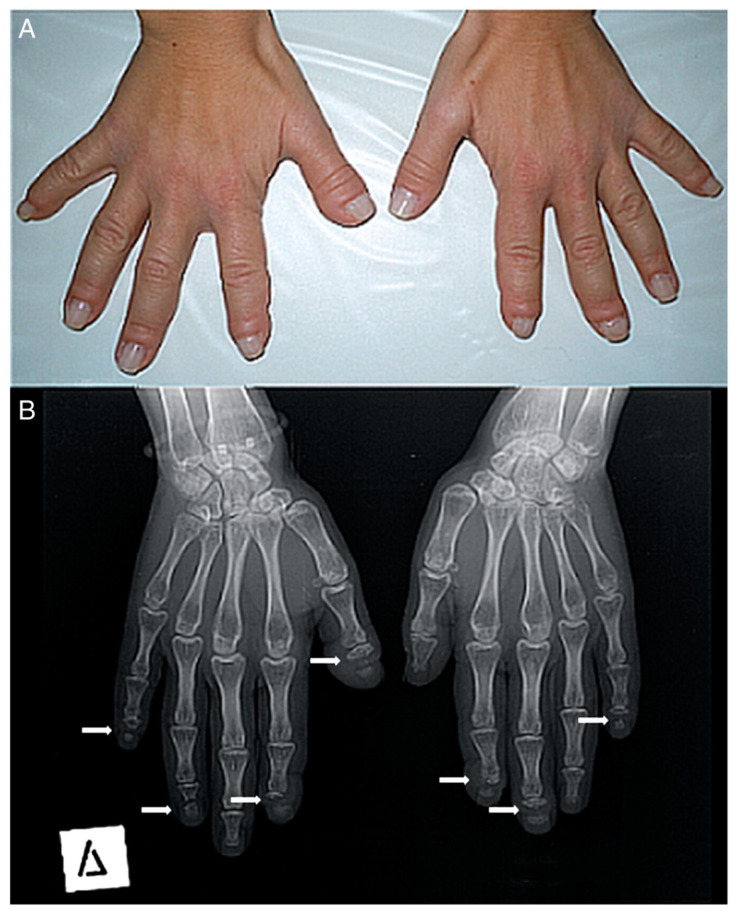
(A) Photograph of a patient’s hands. Many of her fingers are thick (predominately the right thumb) with pseudo-clubbing. (B) Anteroposterior radiograph of a patient’s hands. Osteolysis of the distal phalanges is found in most of the fingers (only the left thumb, the left fourth, and the right third fingers have a normal appearance). In all lesions, osteolysis has a transverse pattern across the width of the terminal phalanx (white arrows).
